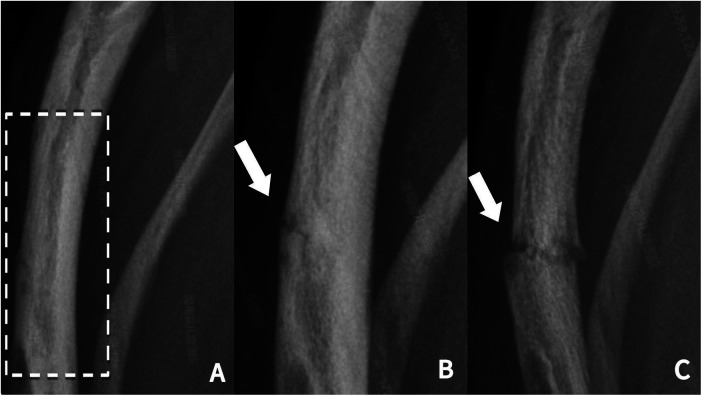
Typical lateral radiographic manifestations of low-density lines in children with ALBT. (A) Initial radiograph showing intact cortical continuity with no identifiable LDL. (B) Follow-up radiograph demonstrating a linear radiolucent band within the anterior tibial cortex (arrow), consistent with LDL appearance. (C) Radiograph obtained approximately 4 months after LDL detection, showing progression to complete tibial fracture at the site of the previously identified LDL (arrow). All three images are lateral views.
